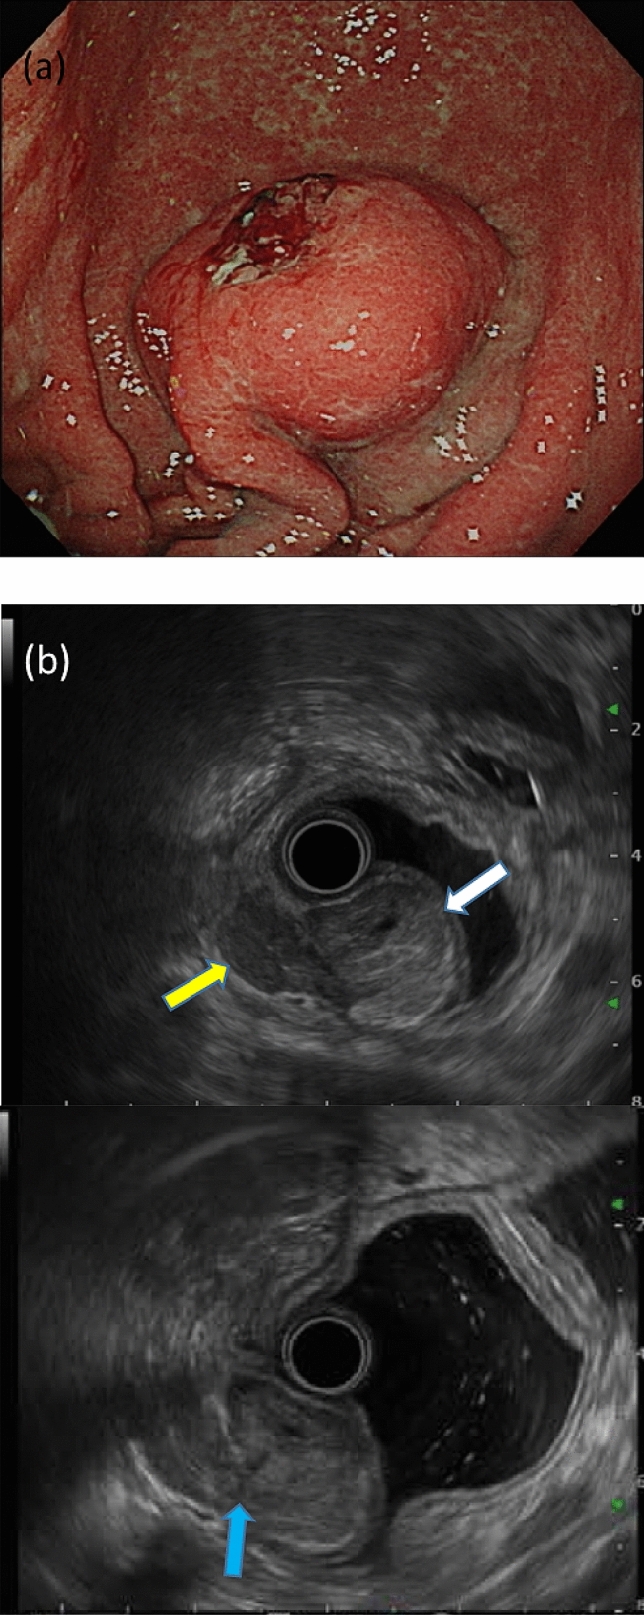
Ultrasound endoscopy suggests the possibility of collision tumor. a Esophagogastroduodenoscopy shows a submucosal tumor-like elevated lesion with delle. b Tumor with the third layer as the main locus (white arrow). Tumor with the fourth layer as the main locus (yellow arrow). The boundary obscure areas of both tumors (blue arrow)
